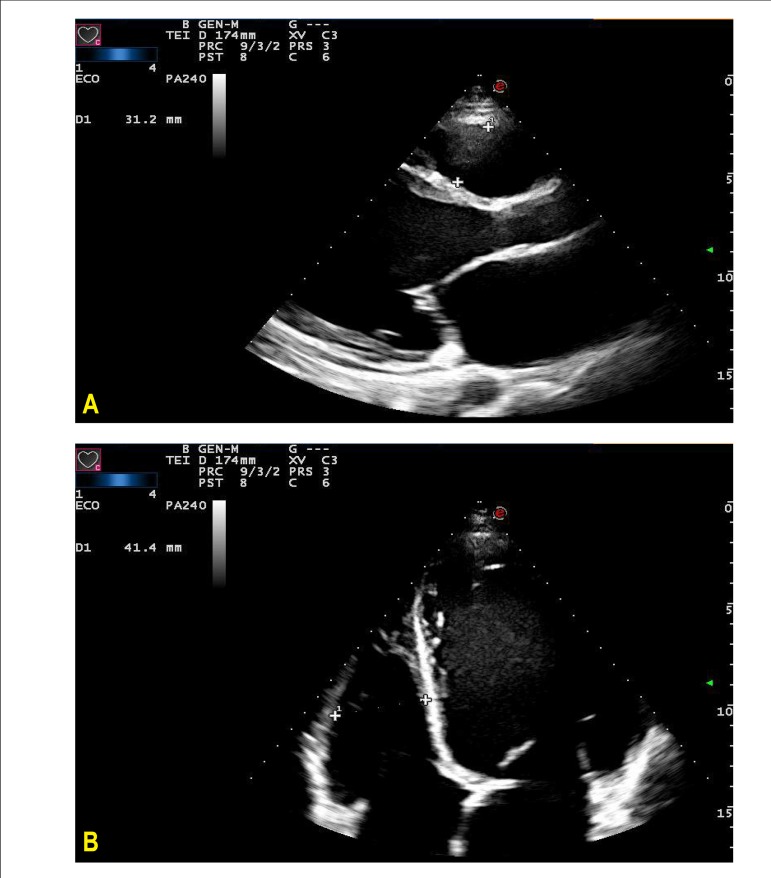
Echocardiogram. A) Longitudinal parasternal view. Enlargement of left
